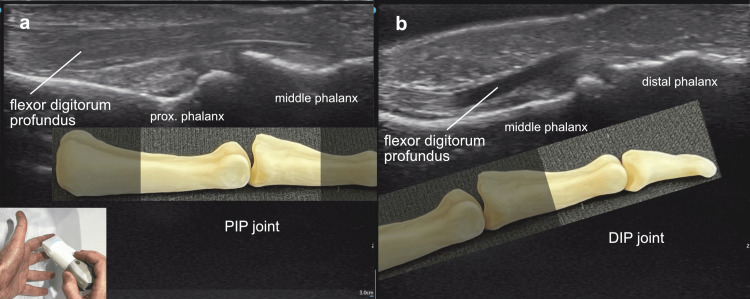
Flexor digitorum profundus Flexor digitorum profundus: (a) Ultrasound scan over the proximal and (b) distal interphalangeal joints of a left index finger in a water bath, the probe marker oriented toward the base of the finger, demonstrated both the flexor tendon and the site of its insertion into the distal phalanx.
